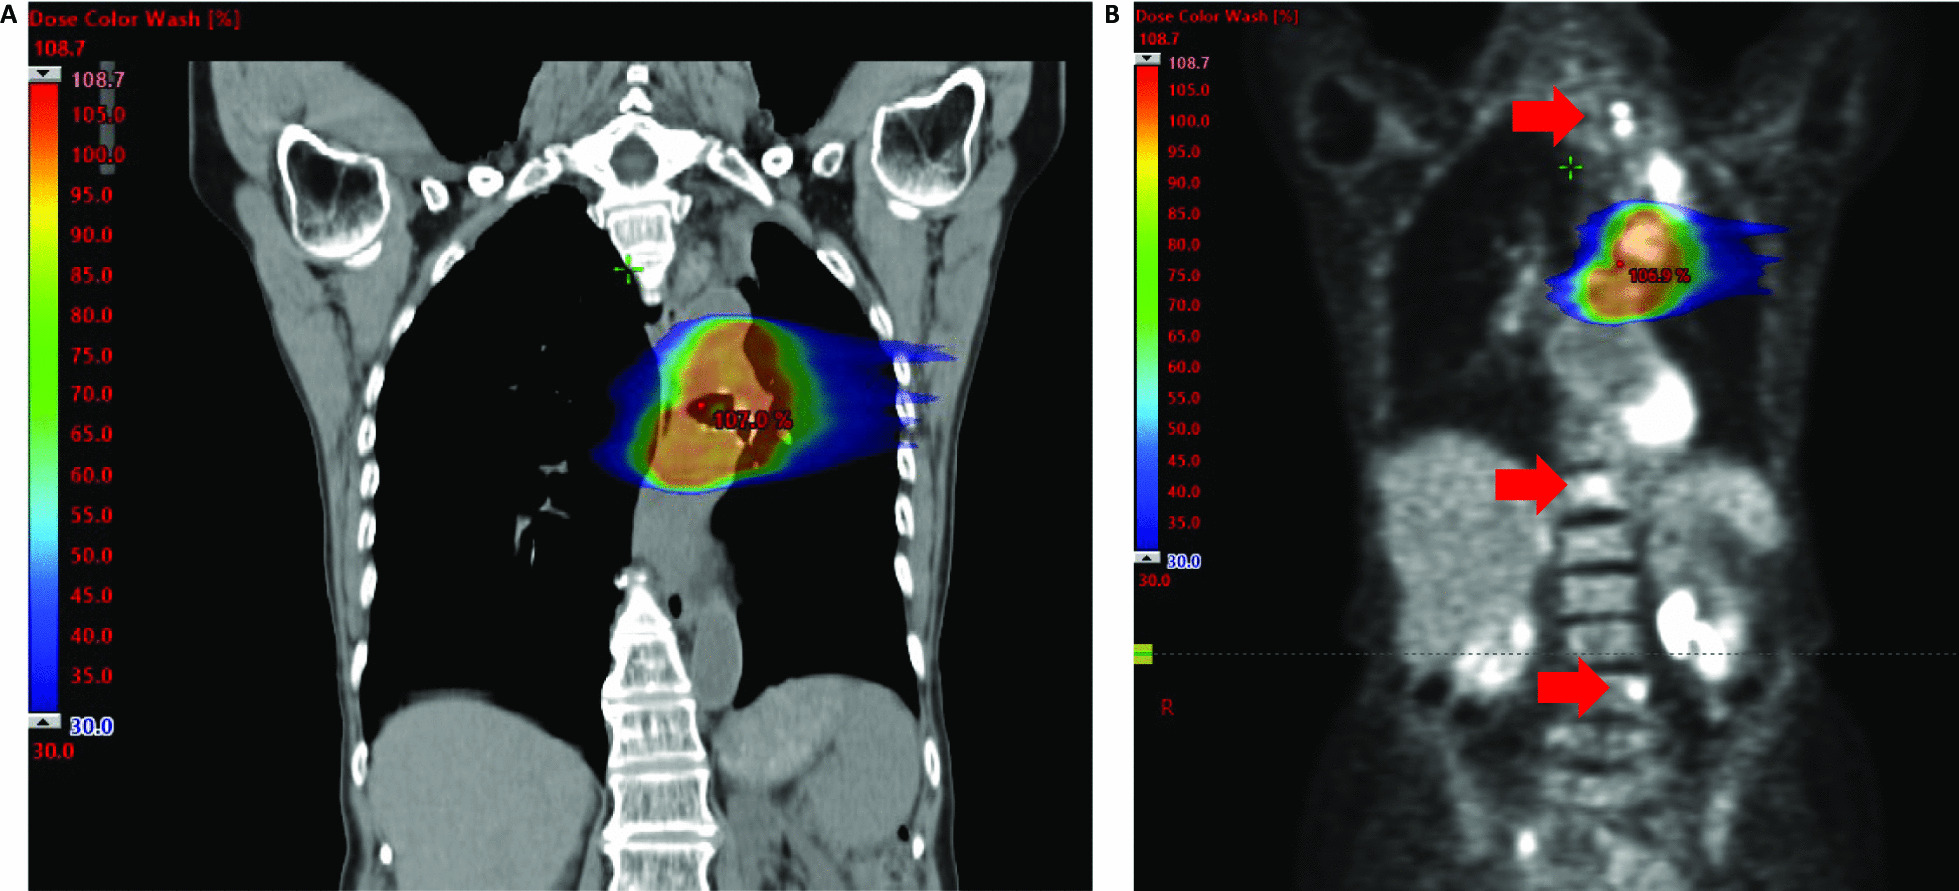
Color wash dose distribution of the applied fractionated radiotherapy with 12 × 3 = 36 Gy. Radiotherapy treatment plan merged with a CT and b PET images in sagittal plane. Color wash is shown as of 30% of the prescribed dose. FDG-positive metastases are marked with red arrows
